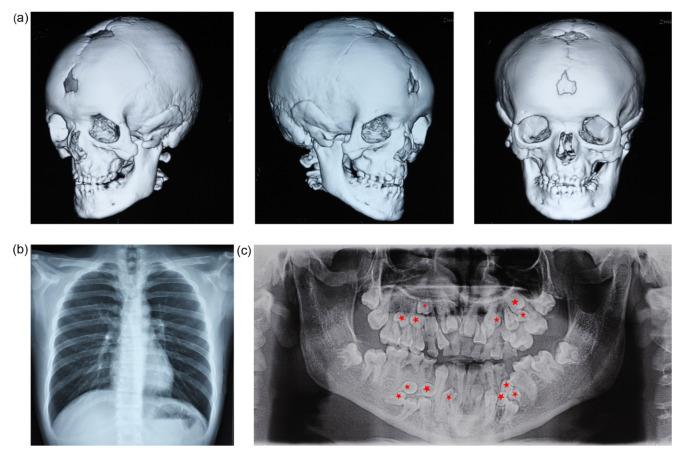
Clinical and radiographic findings of the proband. (a) Different views of CT images. The skull shows non-closing fontanel, frontal bossing, and malocculusion. (b) Chest radiograph demonstrates cone-shaped thorax and hypoplasia of clavicles. (c) Panoramicradiograph shows dental anomalies, including 13 supernumerary teeth (red stars), 14 retained deciduous teeth (51–53, 61–65, 72–74, 82, 84, 85), and 16 failed eruption of permanent teeth (11–13, 21–25, 32–35, 42, 44, 45, 47) (not including the third molars, which have to be followed up).
